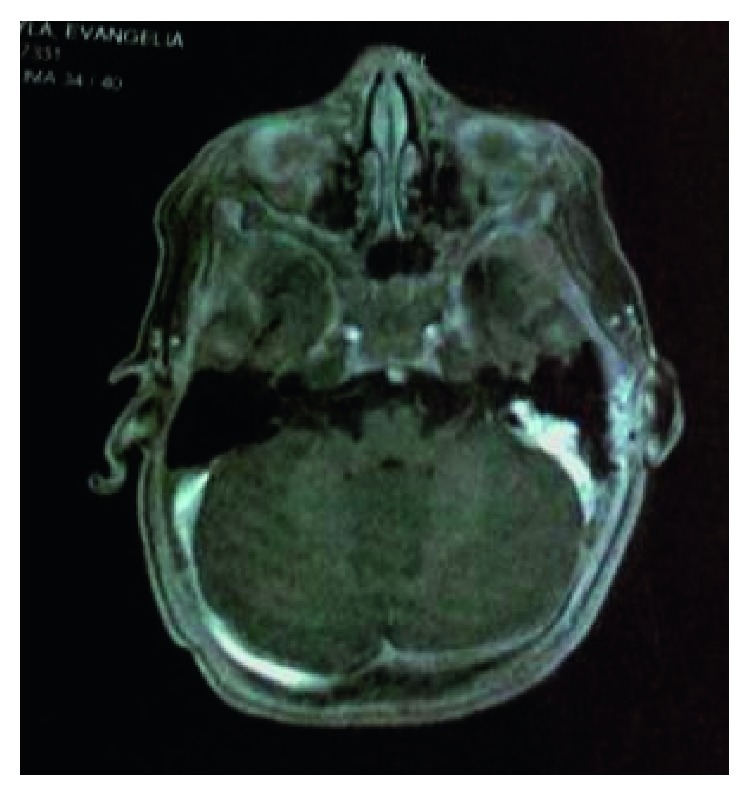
Second MRI (one month later): clear reduction in the area of the pathological signal and of the intake of paramagnetic substance in and around the left temporal bone.
